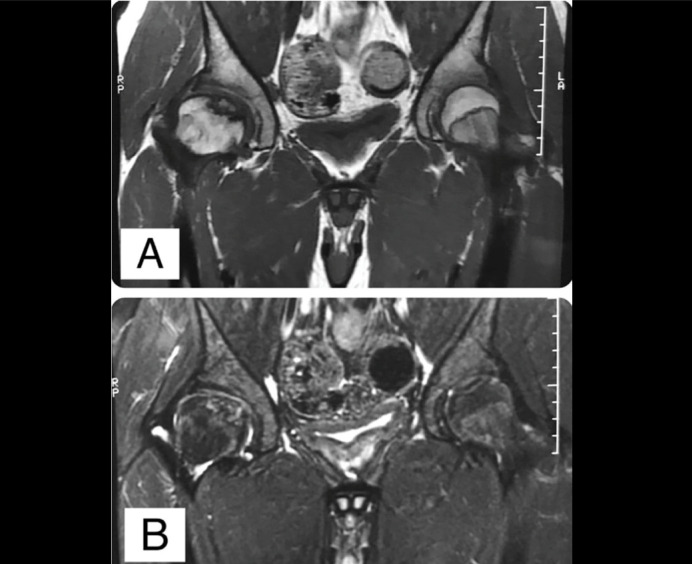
Magnetic Resonance Images (MRI) of both hips in the coronal view. A. T1-weighted MRI showing a non-perfused area surrounding the osteochondral fragment. B. T2-weighted MRI showing intra articular synovitis and edema around the OCD fragment. Note that the articular cartilage has a step-deformity due to a slight elevation of the unstable OCD fragment.
